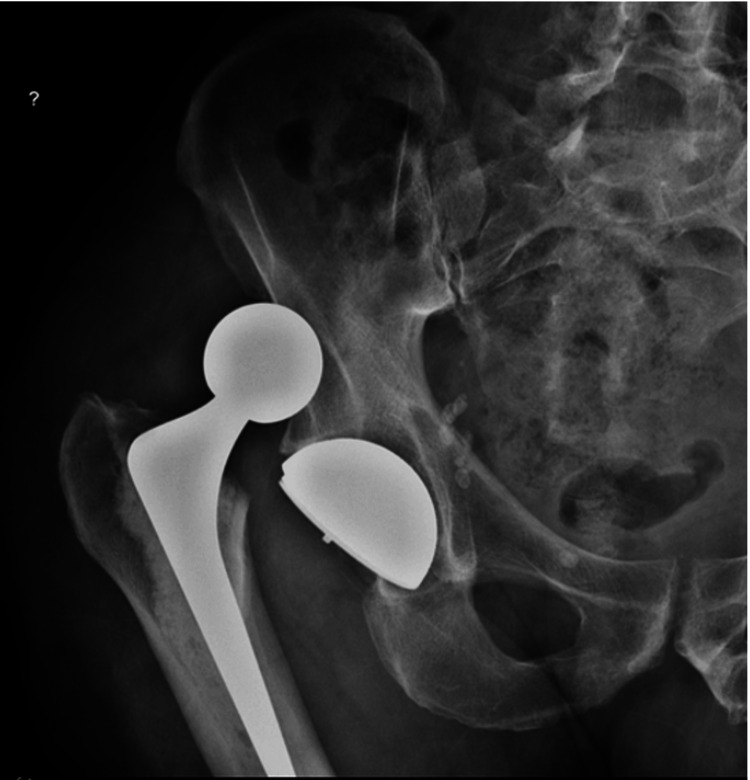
Right pelvic anterior-posterior X-ray showing a dislocation of an implant in a 68-year-old male patient
